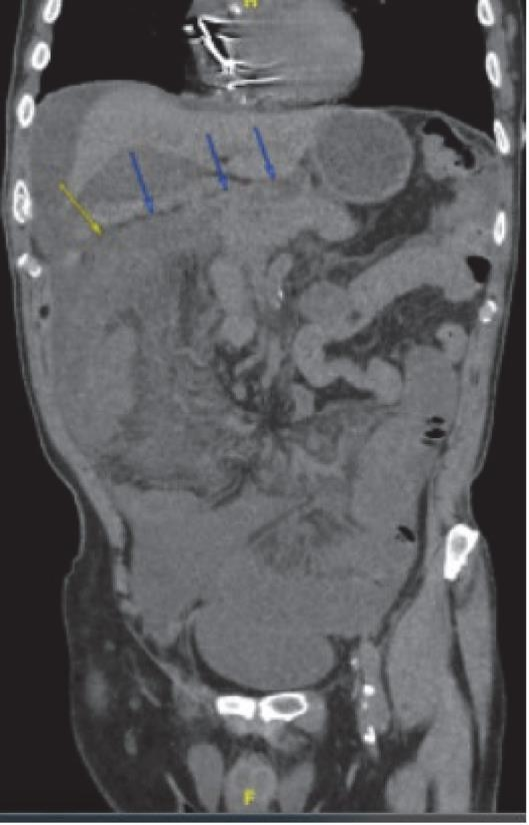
CT showing duodenojejunal junction in the right hemi-abdomen (arrows).
